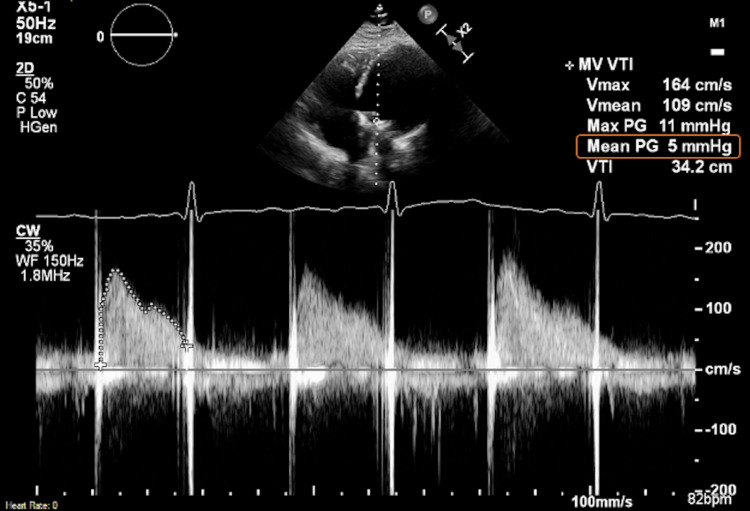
Transthoracic Doppler echocardiogram at six months follow up Transthoracic Doppler echocardiogram at the six months follow up showing a trans-prosthetic mitral valve mean gradient of 5 mmHg at a heart rate of 82 beats per minute.
